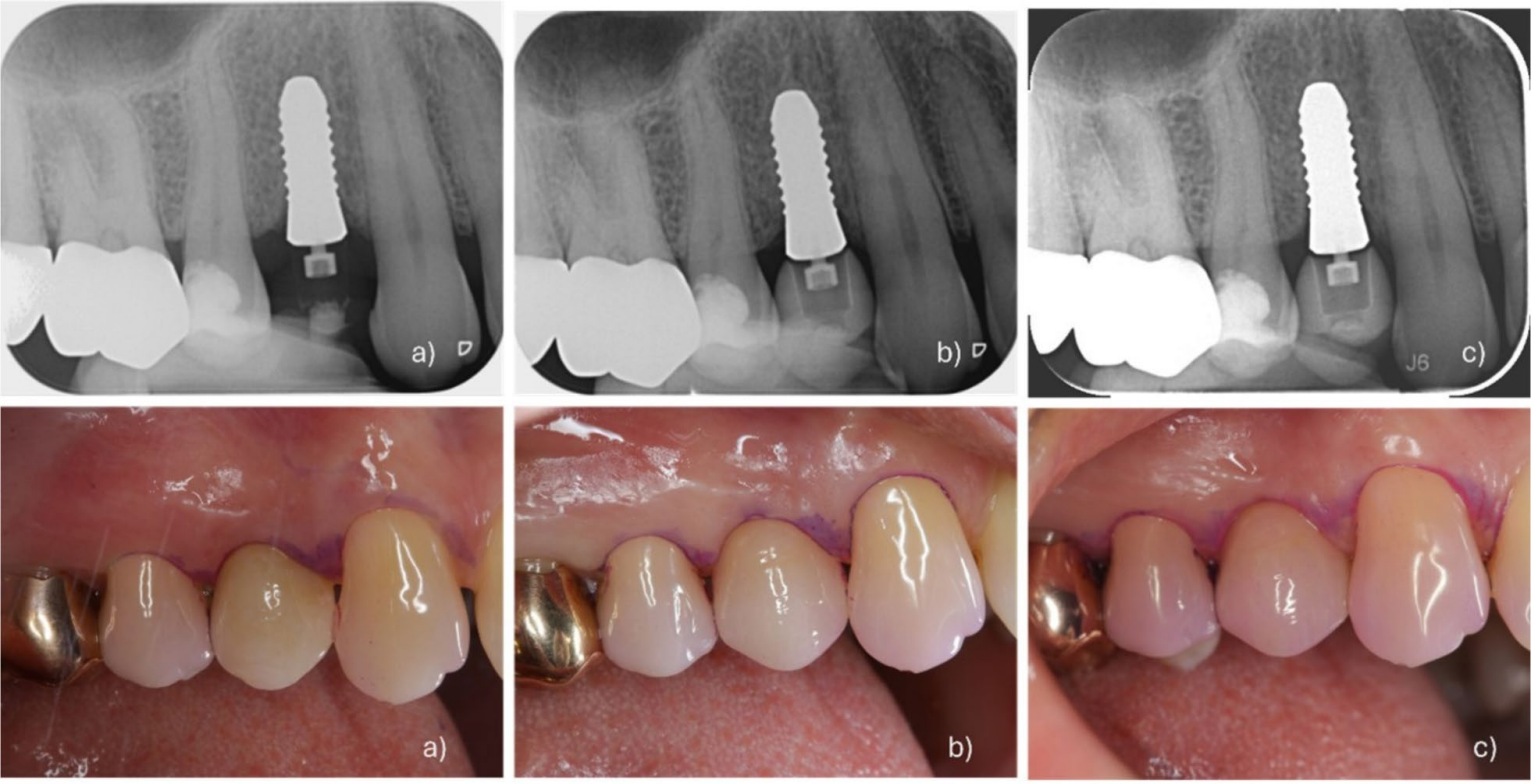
Case of successful implantation and prosthesis placement. X-ray images showing a translucent area around implant abutment is due to the PEKK (Polyetherketoneketone) abutment material, which is radiolucent and thus not visible on x-rays. a X-Ray and intraoral photograph at time of provisional prosthesis placement. b X-ray and intraoral photograph at time of definitive prosthesis placement. c X-Ray and intraoral photograph at 12-month follow-up with stable soft tissue clinical parameters
